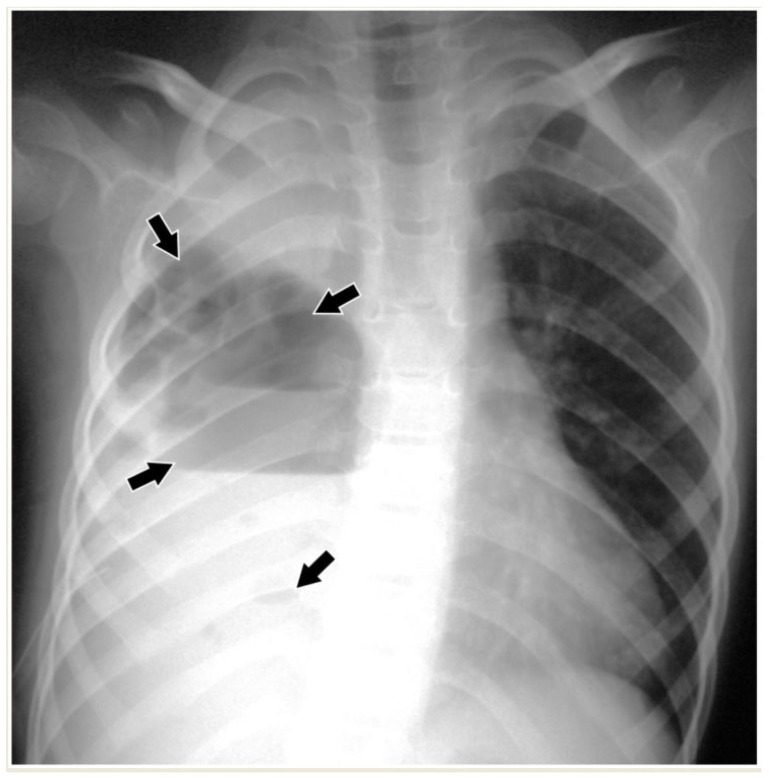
Necrotizing pneumonia in an 8-year-old boy. Chest radiograph shows opacification of the right hemithorax with ipsilateral concave scoliosis and multiple cavities (arrows) with air–fluid levels of variable size.
